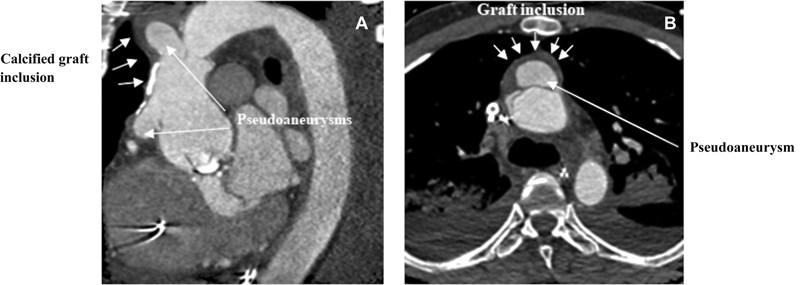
Preoperative computed tomography (CT) scan showing (
